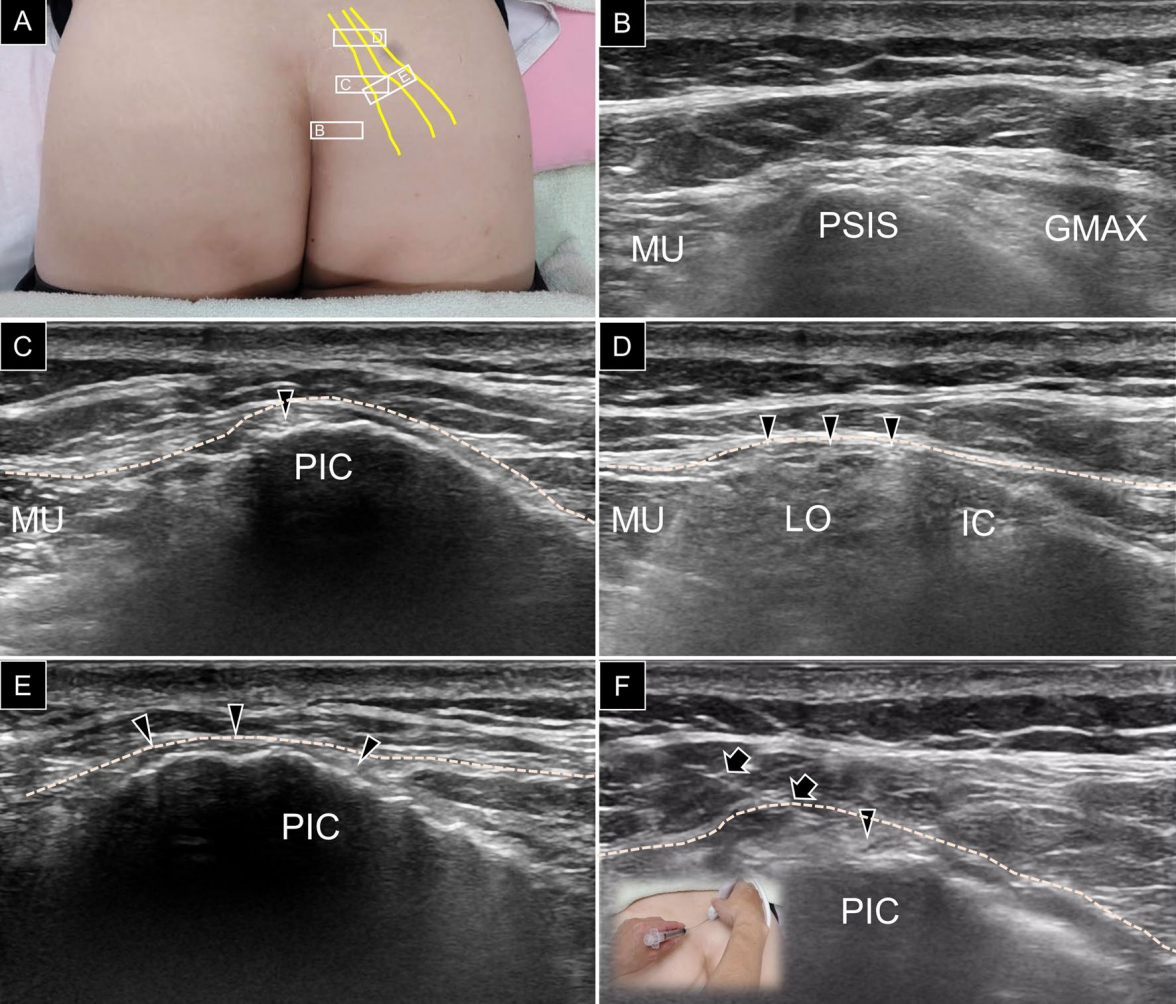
A A, B, C and D inside the squares correspond to probe positioning for the subgraphs of A, B, C and D for scanning of the superior cluneal nerves (yellow lines and black arrowheads). B The probe is placed in the horizontal plane over the posterior superior iliac spine (PSIS) and (C) is then relocated more cranially to see the medial branch of the superior cluneal nerve embedded underneath the thoracolumbar fascia (white dashed line). D Moving the probe more cranially, the superior cluneal nerve is seen over the erector spinae muscle. E Shifting the probe more laterally, the three branches of the superior clunear nerve are identified along the posterior iliac crest (PIC). F Ultrasound guided hydrodissection is performed for the superior cluneal nerves using the in-plane, medial-to-lateral approach. MU, multifidus; GMAX, gluteus maximus; LO, longissimus; IC, iliocostalis; arrows, needle trajectory
